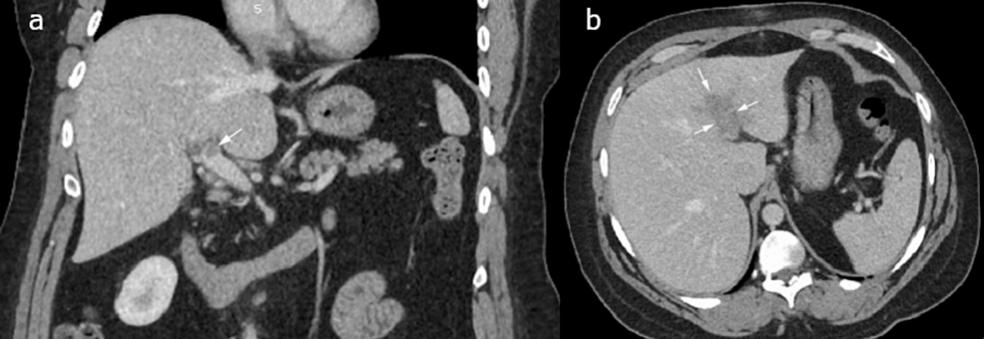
CT scan with contrast at portal venous phase revealed extensive thrombus extending from the main portal vein bifurcation (coronal CT, Figure 1A) into the left portal vein with periportal edema (axial CT, Figure 1B)
